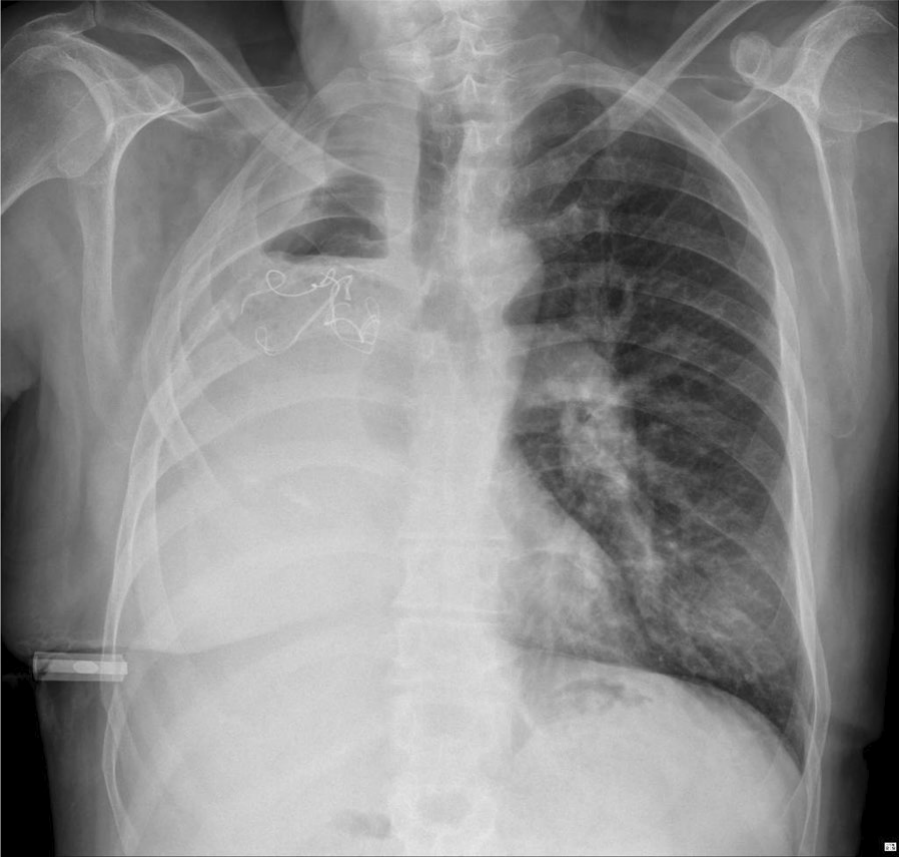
Chest X-ray showing right hemithoracic opacity with thread-like calcifications
